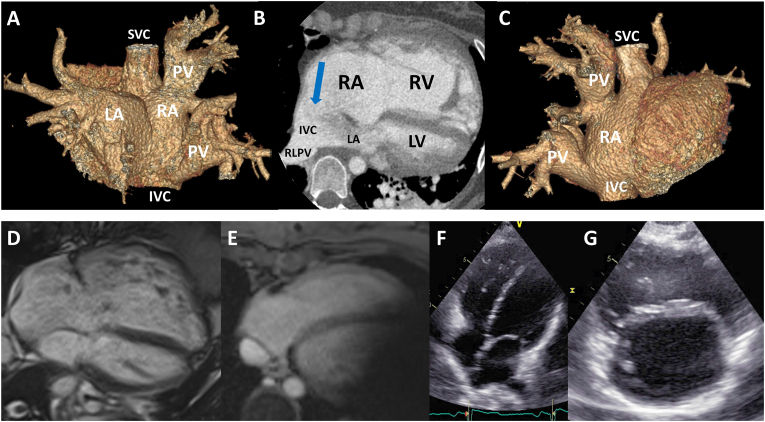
Posterior (A) and anterior (C) 3D reconstruction of CT of atria and anomalously connected right pulmonary veins draining into SVC, RA and IVC. (B) Modified 4CH view showing the ISV-ASD (blue arrow). (D) Cardiac magnetic resonance (CMR), 4CH showing the dilated RV. (E) CMR of RV post-repair with artifact of S-ICD. (F) and (G) Post-repair 4CH and PSAX showing the reduced RV size. Abbreviations: IVC: inferior vena cava, LA: left atrium, RV: right ventricle, LV: left ventricle, PV: pulmonary veins RA: right atrium, RLPV: right lower pulmonary vein, other see Fig. 1. . (For interpretation of the references to color in this figure legend, the reader is referred to the Web version of this article.)
